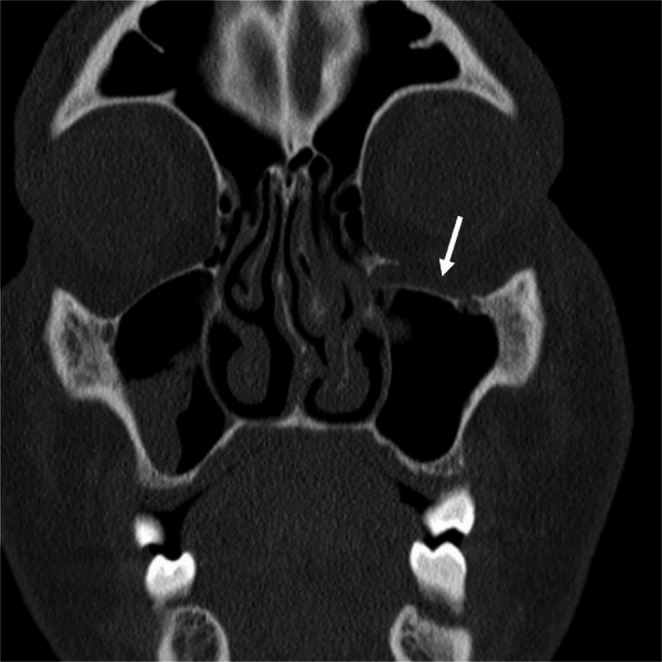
46-year-old male status post assault. Coronal CT of the face demonstrates a left orbital floor fracture (arrow) which was not described in the original outside report. The fracture was treated non-operatively
